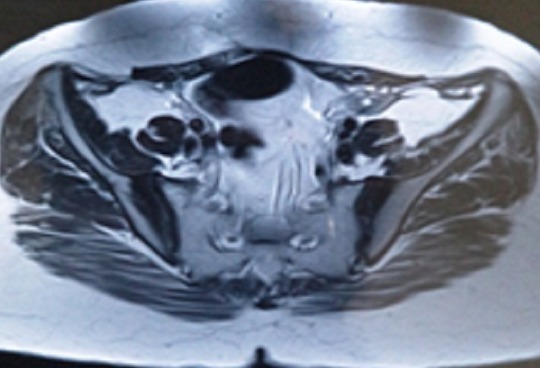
MRI on T2 sequence showing the condensation of the iliac side of the sacro iliac joints with no involvment of the joint nor the soft tissues
